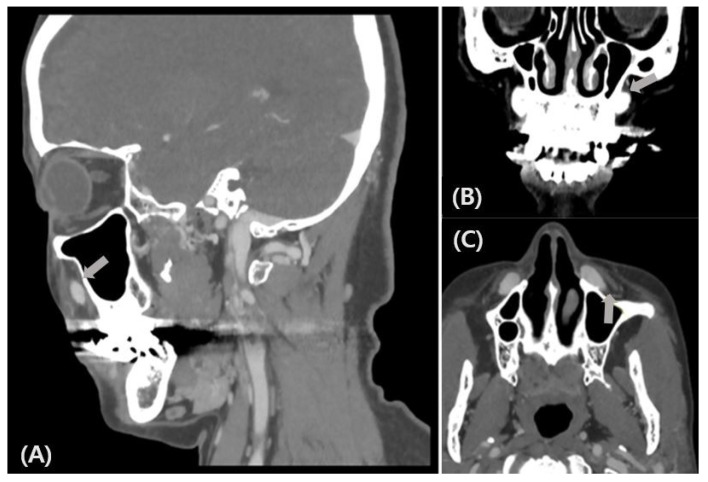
Sagittal (A), coronal (B) and axial (C) facial computed tomography images in a 49-year-old woman show that the left infraorbital nerve is intact around the infraorbital foramen and travels to the peripheral side of the foreign body. A prominent nerve contact or compression is not apparent, but the contact of the peripheral branch of the left infraorbital nerve (arrows in A–C) is possible.
